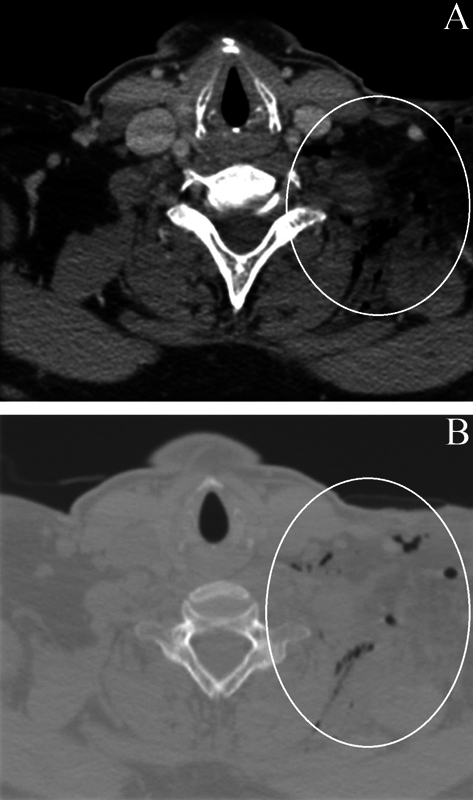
Postoperative computed tomography in axial section in bone window in A and soft-tissue window in B demonstrating densification of the deep planes of the surgical bed, as well as local unorganized liquid slides and sparse foci of gaseous component (white circles).
